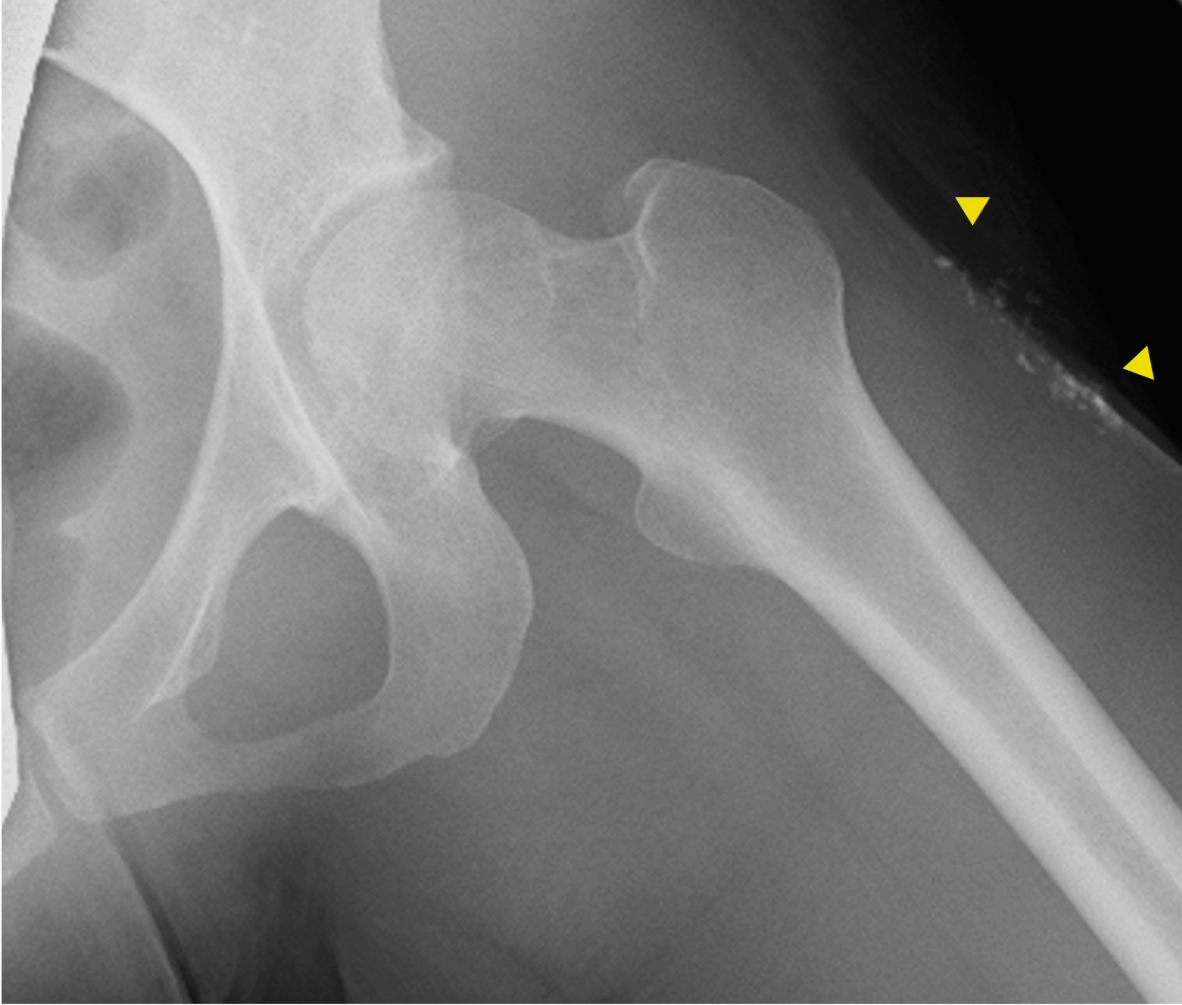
Radiograph of the left thigh showing subcutaneous calcinosis (arrows).
